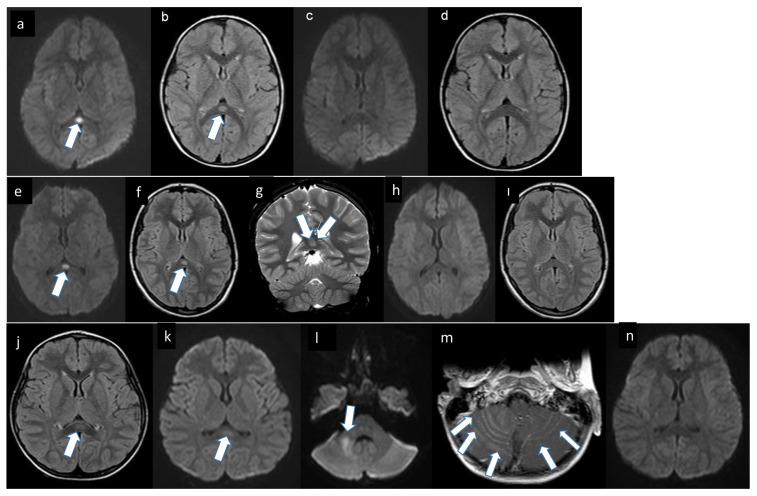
(a–n) legend: a: Diffusion-weighted image of the patient shows restricted diffusion in the splenium of the corpus callosum *(arrow)*. b: FLAIR image demonstrates high signal intensity in the splenium of the corpus callosum*(arrow)*. c,d:On follow-up MRI, the lesion in the splenium of corpus callosum was totally resolved. e: Diffusion-weighted image of the patient reveals restricted diffusion in the splenium of the corpus callosum *(arrow)*. f: Axial plane FLAIR and g: coronal plane T2 weighted images demonstrate high signal lesion in the splenium of the corpus callosum compatible with edema *(arrow)*. h,ı:On follow-up MRI, the lesion in the splenium of corpus callosum was totally gone. j: FLAIR image of brain MRI shows high signal intensity in the splenium of the corpus callosum *(arrow)*. k,l: Diffusion-weighted image shows restricted diffusion in the splenium of the corpus callosum and right side of the middle cerebellar peduncle. m: Post contrast T1 weighted image shows the leptomeningeal enhancement of the cerebellar folia more prominent on the right side than the left compatible with cerebellitis (arrows). n: On the follow-up MRI, the DWI image reveals the restricted diffusion in the splenium of corpus callosum was totally gone.
